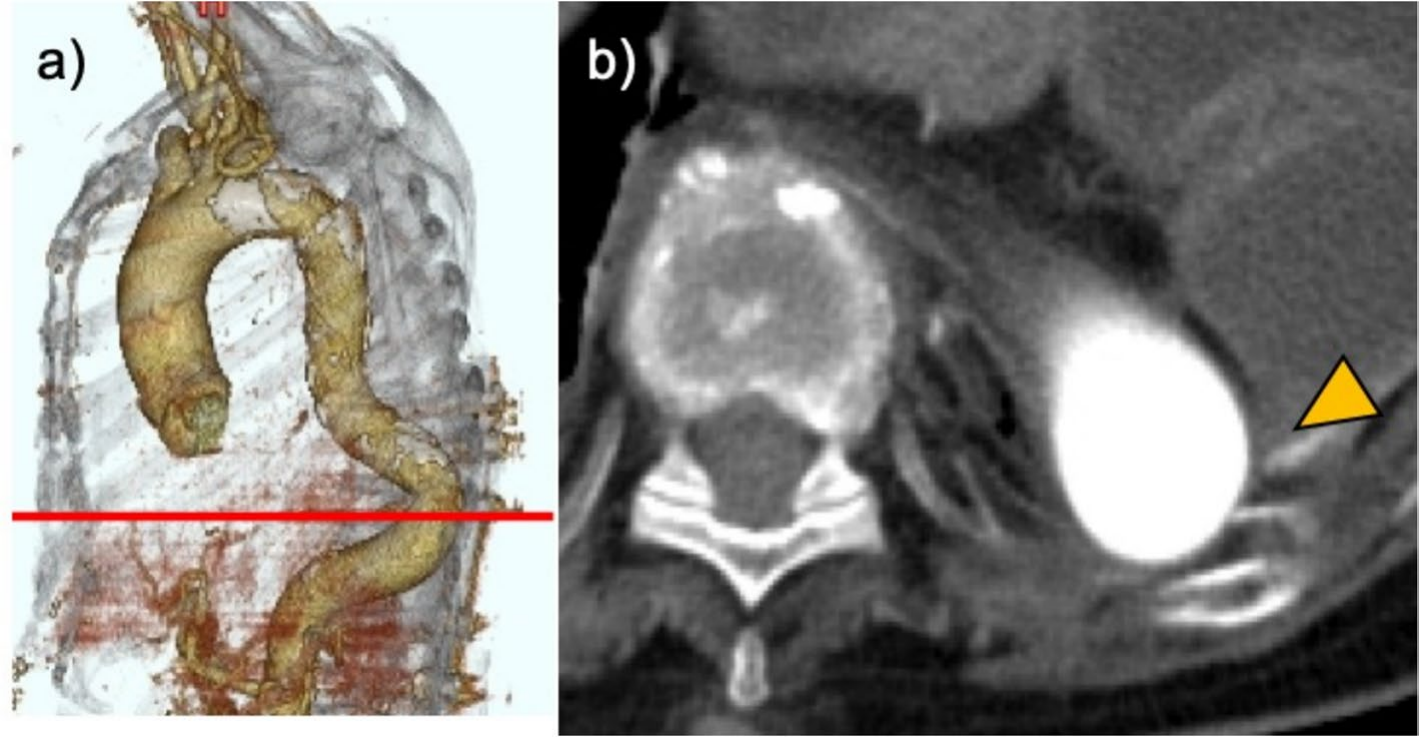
CT image of the injured area: a aortic tortuosity. b Displacement of the fractured end of the left 10th rib, which is in close proximity to the tortuous part of the aorta. CT, computed tomography
